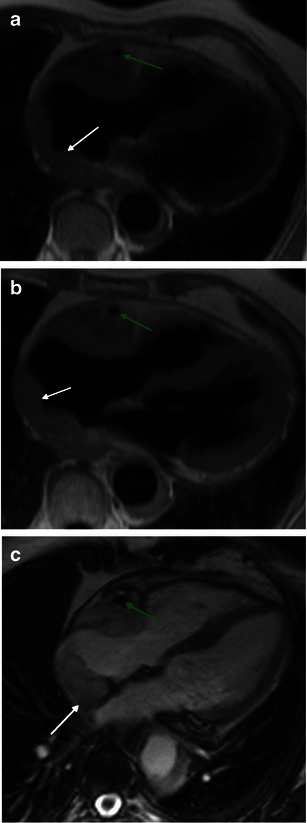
Axial HASTE (a), T1 (b) and four-chamber SSFP (c) cardiac MR images in a 71-year-old female, showing hypointense soft tissue infiltration of the right atrium and right atrio-ventricular sulcus (white arrrow), periarterial infiltration of the right coronary artery (green arrow) and periaortic infiltration (white arrow)
